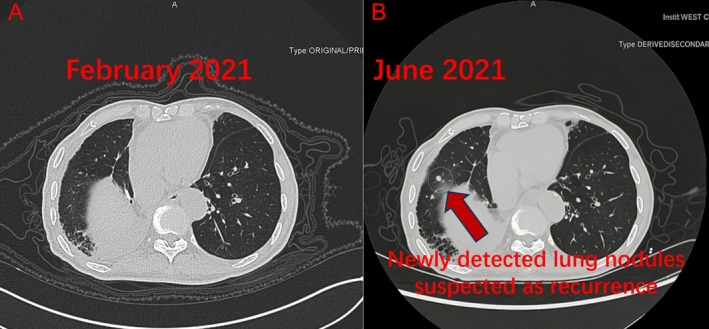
Computed tomography images of the octogenarian patient showing newly detected lung nodules in the right lung lobe suspected to be recurrent lesions. (A) No sign of recurrence in February 2021. (B) Newly detected lung nodules suspected as recurrence.
